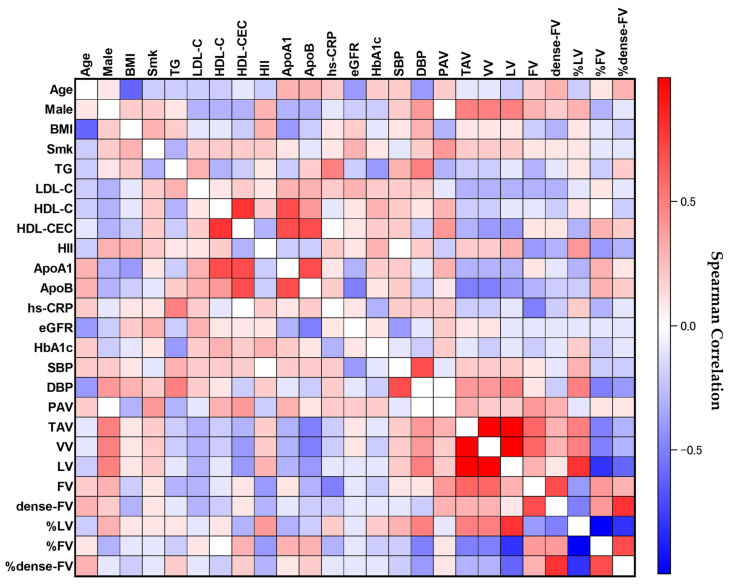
Correlations between IVUS-derived measures and clinical demographics, including HDL functionality. The heat map explains correlations between IVUS-derived measures and clinical demographics, including HDL functionality. Darker red indicates stronger positive correlations, and darker blue indicates stronger negative ones. ApoA1 = apolipoprotein A-1, ApoB = apolipoprotein B, BMI = body mass index, DBP = diastolic blood pressure, dense-FV = dense fibrous volume, eGFR = estimated glomerular filtration rate, FV = fibrous volume, HbA_1c_ = glycosylated hemoglobin, HDL-C = high-density lipoprotein cholesterol, HDL-CEC = HDL-mediated cholesterol efflux capacity, HII = HDL inflammatory index, hs-CRP = high sensitivity C-reactive protein, HT = hypertension, IVUS = intravascular ultrasound, LDL-C = low-density lipoprotein cholesterol, LV = lipid volume, PAV = percent atheroma volume, SBP = systolic blood pressure, Smk = smoking, TAV = total atheroma volume, TG = triglyceride, VV = vessel volume, %dense-FV = percentage of dense fibrous volume, %FV = percentage of fibrous volume, %LV = percentage of lipid volume.
